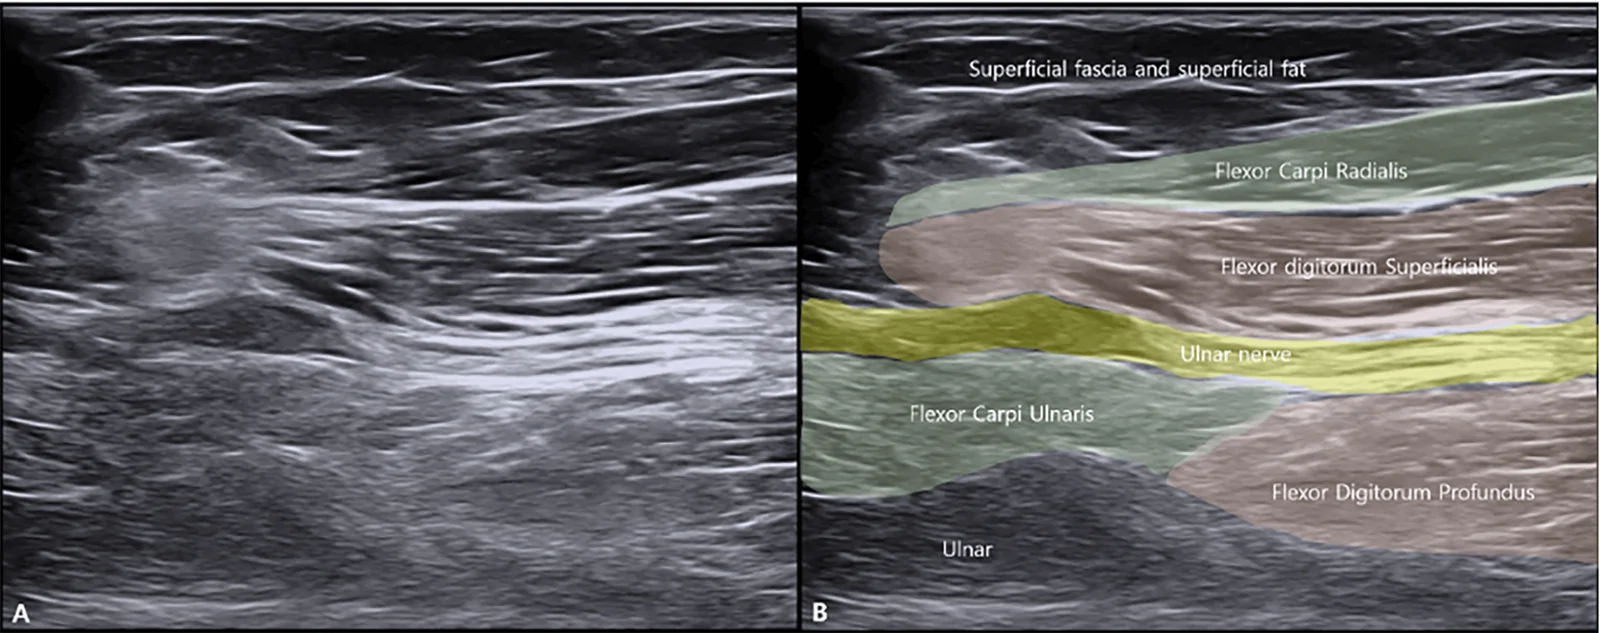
Aberrant gliding of the FDS during dynamic long-axis ultrasound Long-axis ultrasound view of the FDS in the proximal forearm. At the indicated site, approximately 3-4 cm distal to the medial epicondyle, tissue associated with the FDS demonstrated irregular, jerky motion during active finger flexion and extension. This motion abnormality was temporally associated with the patient's triggering sensation and supported the presence of a proximal symptomatic site. FDS, flexor digitorum superficialis Image courtesy: Yonghyun Yoon and King Hei Stanley Lam
